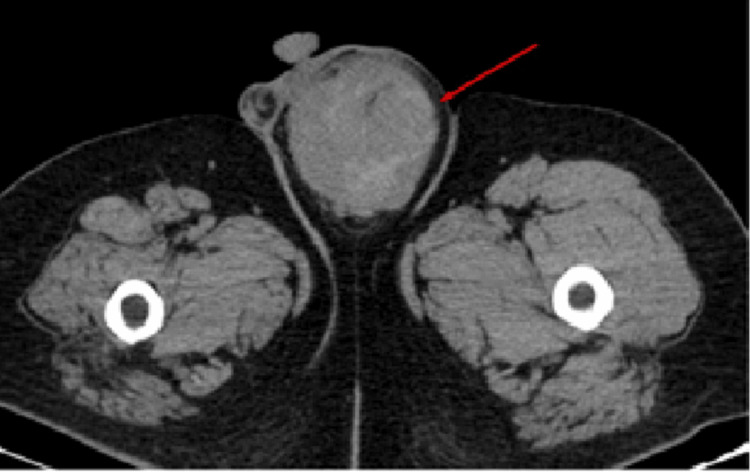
Axial section of non-contrast CT shows a well defined heterodense lesion in the left para testicular region (red arrow). No evidence of any calcific specs seen.
